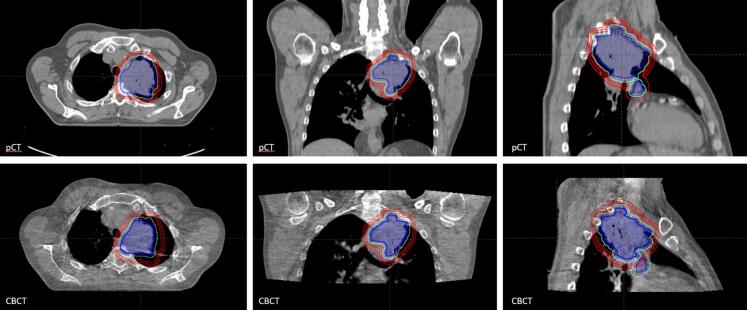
Transverse, coronal and sagittal view of the target volumes with the different margins for the primary tumor on the planning CT (top) and CBCT of a fraction (bottom). The GTV and CTV are adjusted to meet the anatomy of the CBCT, the PTVs are copied rigidly from the planning CT. Red: PTV of primary tumor with different margins (8 mm, 5 mm, 2 mm). Green: PTV of the nodes (5 mm margin). Light blue: CTV. Dark blue: GTV. pCT: planning CT, CBCT: cone-beam CT.
